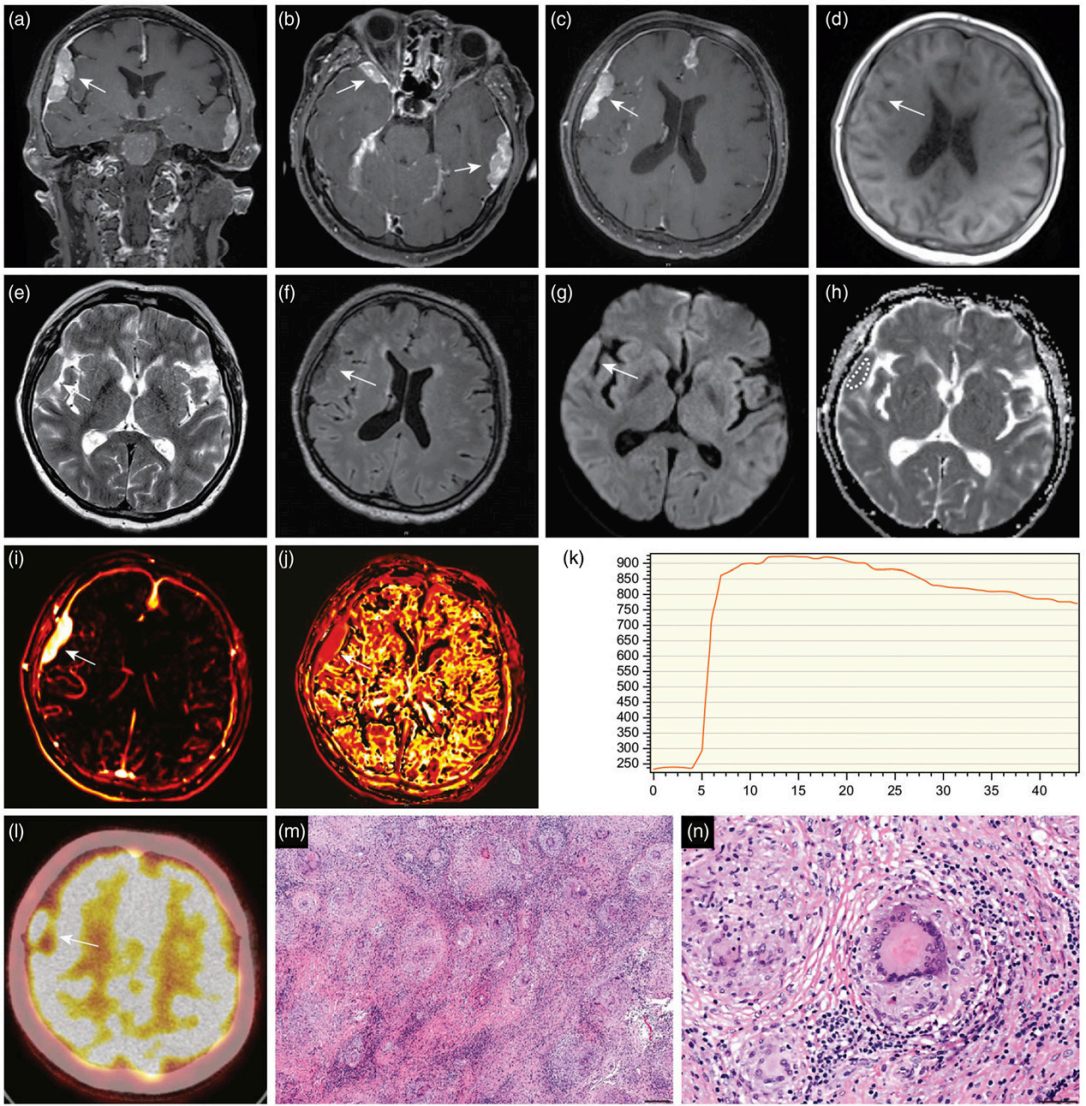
Multiparametric magnetic resonance imaging (MRI) of the head (A)–(K), fused ^18^F-fluorodeoxyglucose positron emission tomography/computed tomography (^18^F-FDG-PET/CT) (l) and biopsy (M), (N) performed in a patient with neurosarcoidosis. Coronal and axial T1-weighted sequences after gadolinium administration show homogenously enhancing, extraaxial lesions (A–C, white arrows). The dural lesions have a solid appearance and low signal on the axial precontrast T1-weighted sequence (D, white arrow), intermediate signal on the axial T2-weighted sequence (C, white arrow), and intermediate/low signal on the fluid attenuated inversion recovery (F, white arrow). Axial diffusion-weighted imaging (b = 1000 s/mm^2^) (G, white arrow) shows a high signal intensity in lesions with a corresponding low apparent diffusion coefficient value (0.8 × 10^−3^mm^2^/s) (H). Dynamic contrast-enhanced MRI (DCE-MRI) derived the transfer constant (*K*^
